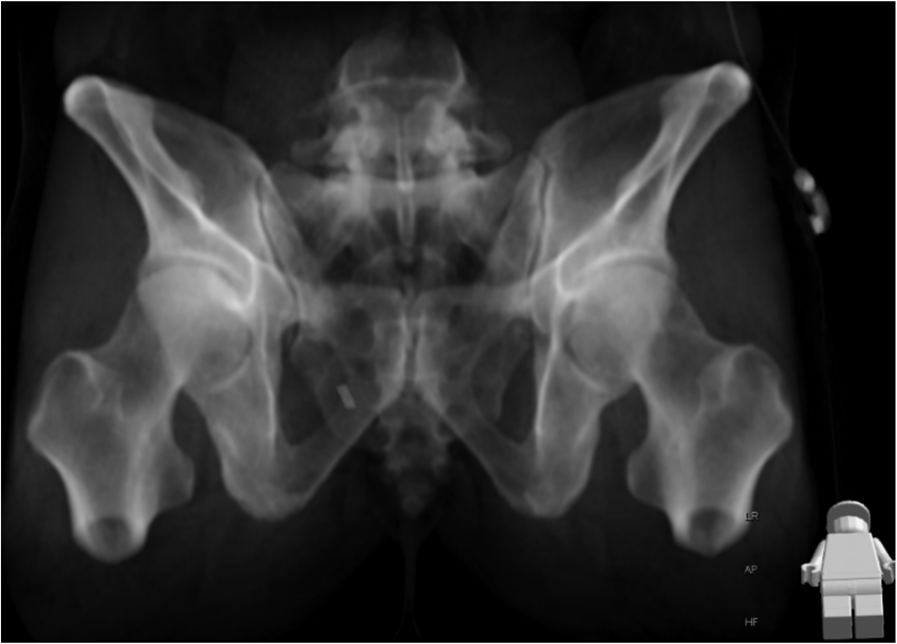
Reconstruction of an outlet ap-view from a full body CT scan using maximum intensity projections (MIP)
